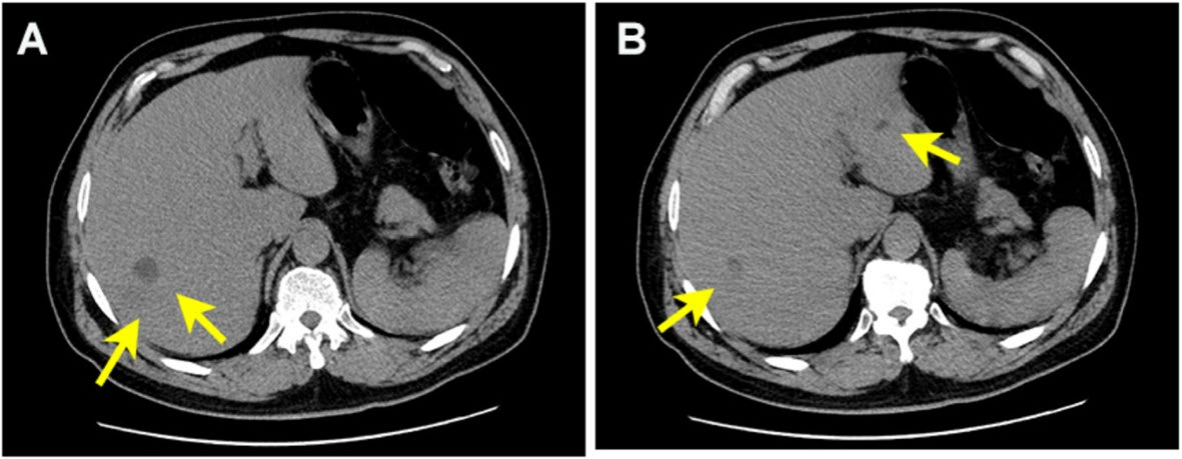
A and B Abdominal computed tomography scan revealed a patchy, slightly low-density area in the lower right posterior lobe of the liver (*arrows*), with an area of about 55 × 45 mm
